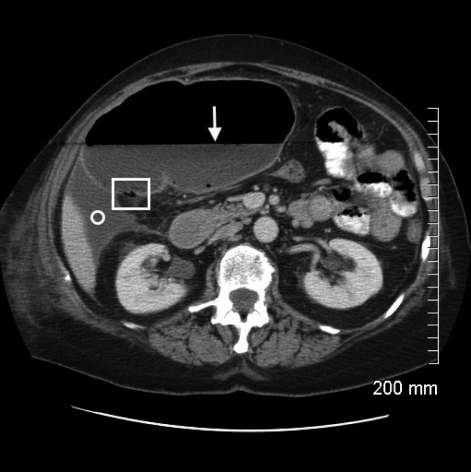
Axial CT image with intravenous and oral contrast through the level of the mid-abdomen demonstrates massive dilatation of the cecum. There is a prominent air-fluid level (white arrow) and multiple locules of air are noted in the dependent portion of the wall consistent with pneumatosis intestinalis (square). However, no extraluminal free air is present to suggest frank perforation and the wall of the cecum continues to enhance uniformly. The associated perihepatic free fluid (circle) is consistent with a high-grade type of intestinal obstruction.
